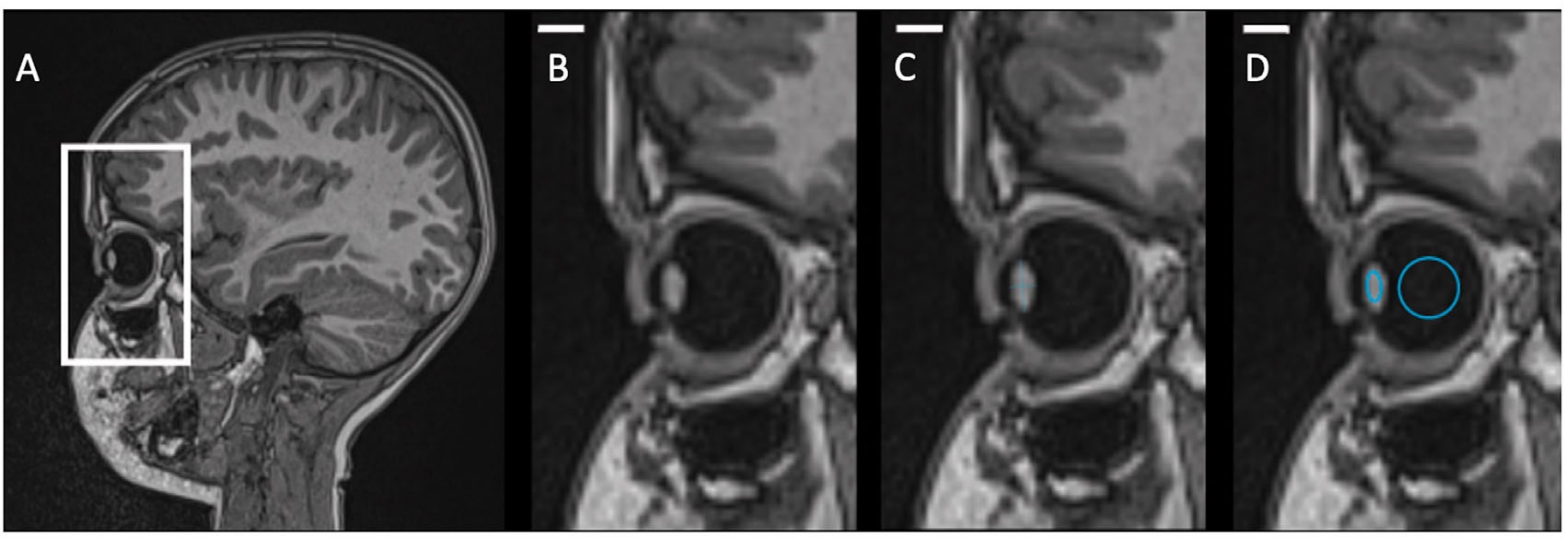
Representative example of an unenhanced T1-weighted 3D MPRAGE brain image in the sagittal plane of a 6-year-old male patient (A). The right eye is magnified (bar ≙ 7 mm) (B). Lens measurements were performed (C) as well as the region of interest measurement of the mean signal intensity were drawn on the lens and vitreous body (D).
